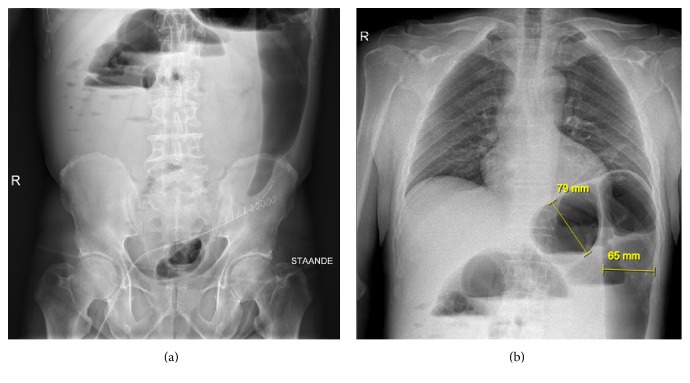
Plain X-ray in standing position showing aerocoly in the epigastric region and the splenic flexure. No evidence of free air under the diaphragm.
